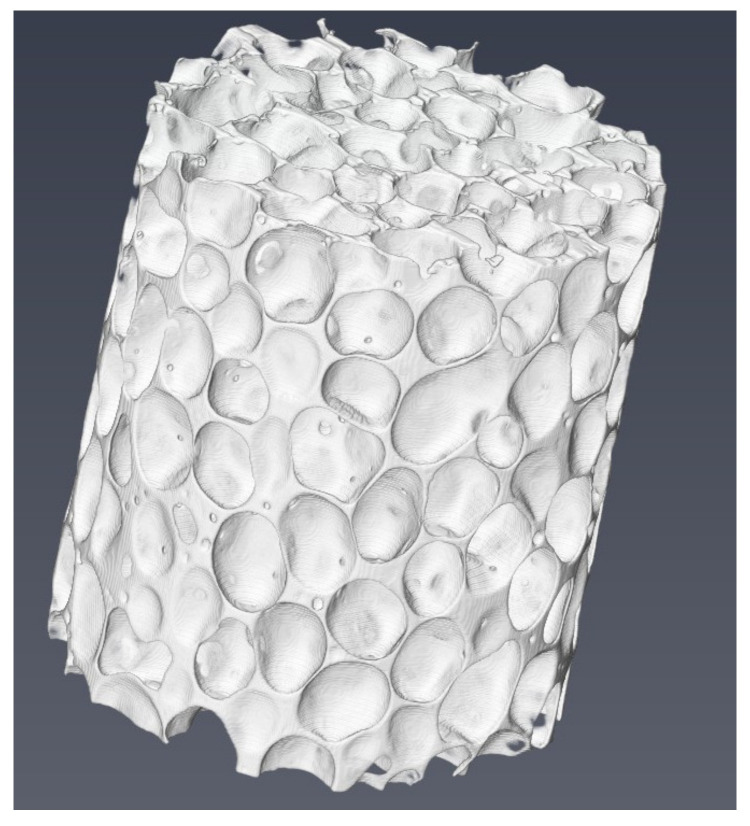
Three-dimensional modeling of aluminum foam based on CT scanning tomography technology.
